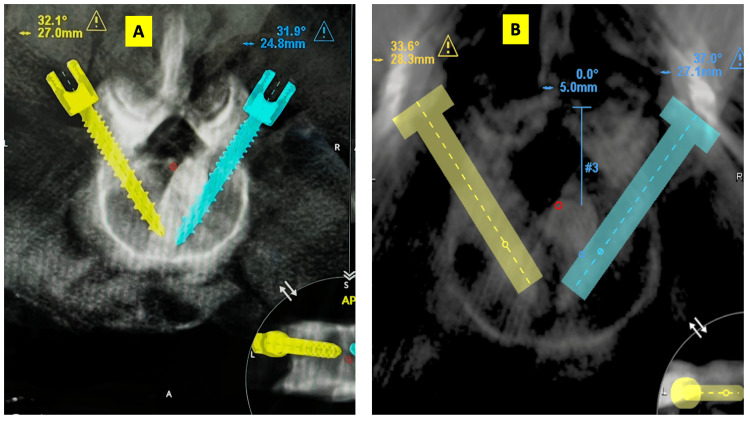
Image showing pre-operative planning and post-operative parameter acquisition from CT scans uploaded into the Mazor X software. (1A) Planned trajectory coordinates noted from the robot console. The yellow and blue screws represent the planned right and left screw trajectories, respectively. (1B) Post-operative CT assessment. Yellow and blue virtual cylinders are placeholders used to measure the angles within the software. These templates are superimposed over the visible screws in the post-operative scan so the final parameters can be recorded.
